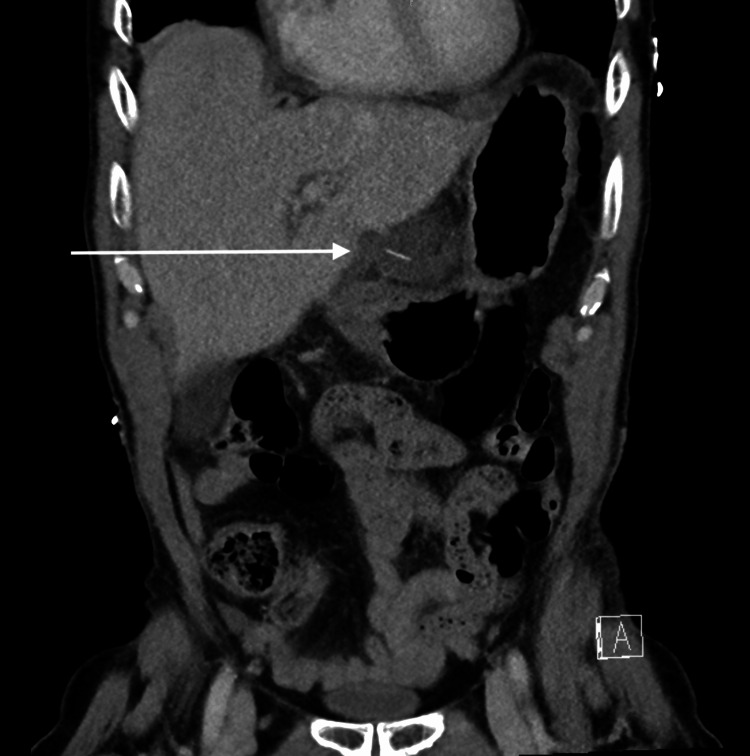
Computed tomography coronal view. The arrows point to a hypodense collection within the lesser sac, with the fish bone at the center appearing to be 25 mm away from the lesser curvature of the stomach.
